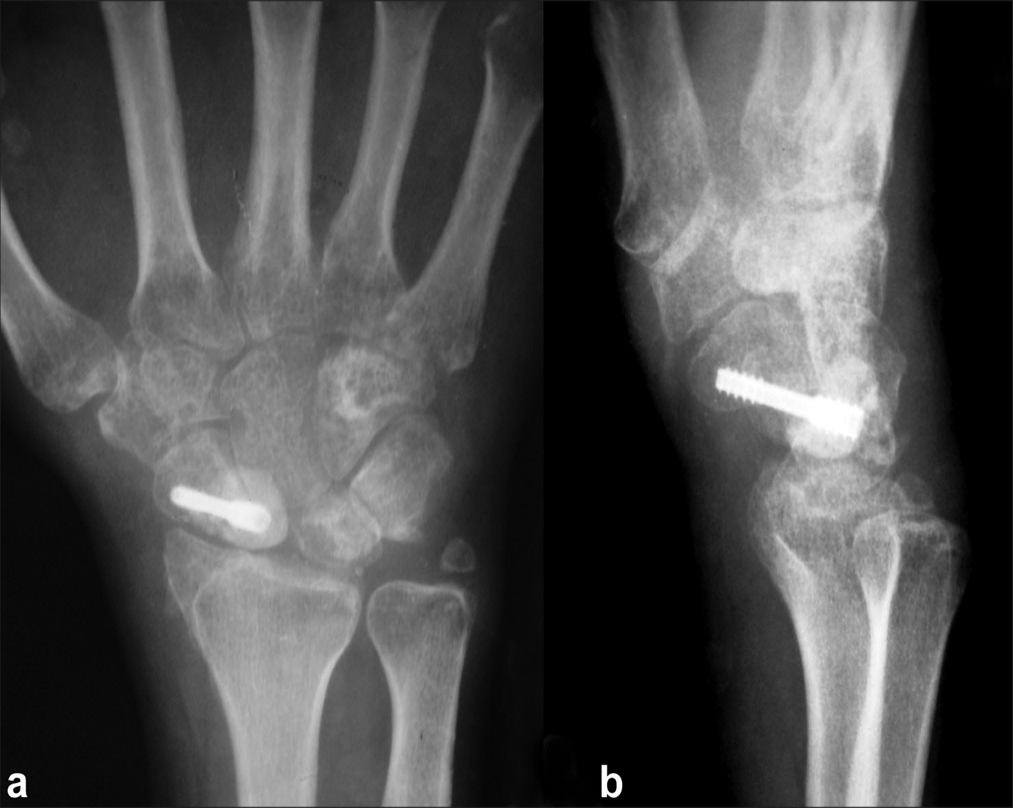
Follow-up anteroposterior (a) and lateral (b) radiograph showing the healed scaphoid fracture. The carpal bones are in acceptable alignment
